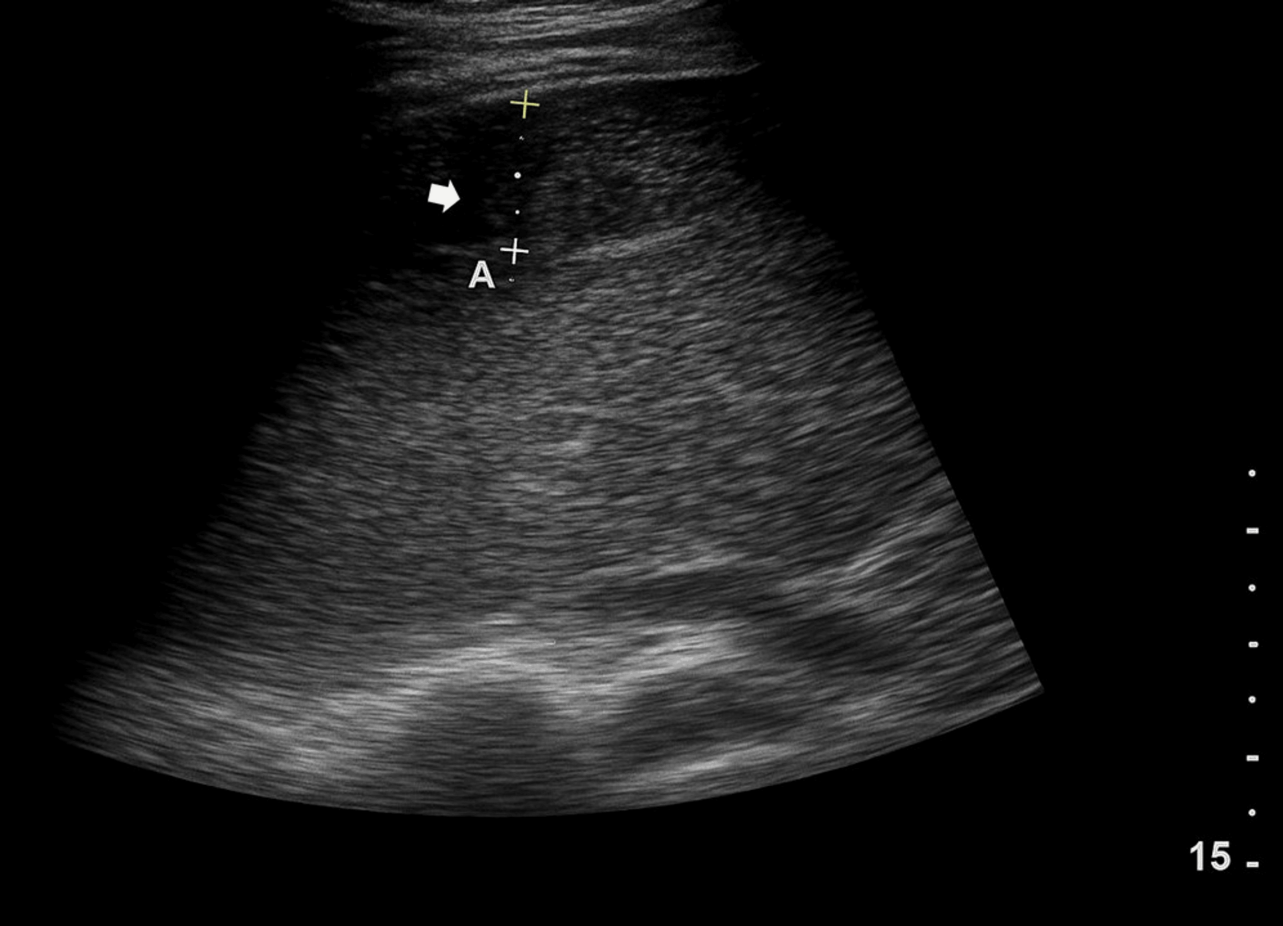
Abdominal ultrasonography showing a well-defined heterogeneous subcapsular collection (white arrow) over the anterior, posterior, and right lateral aspects of the right hepatic lobe, measuring approximately 34 mm in maximal thickness, consistent with a subcapsular hematoma.
